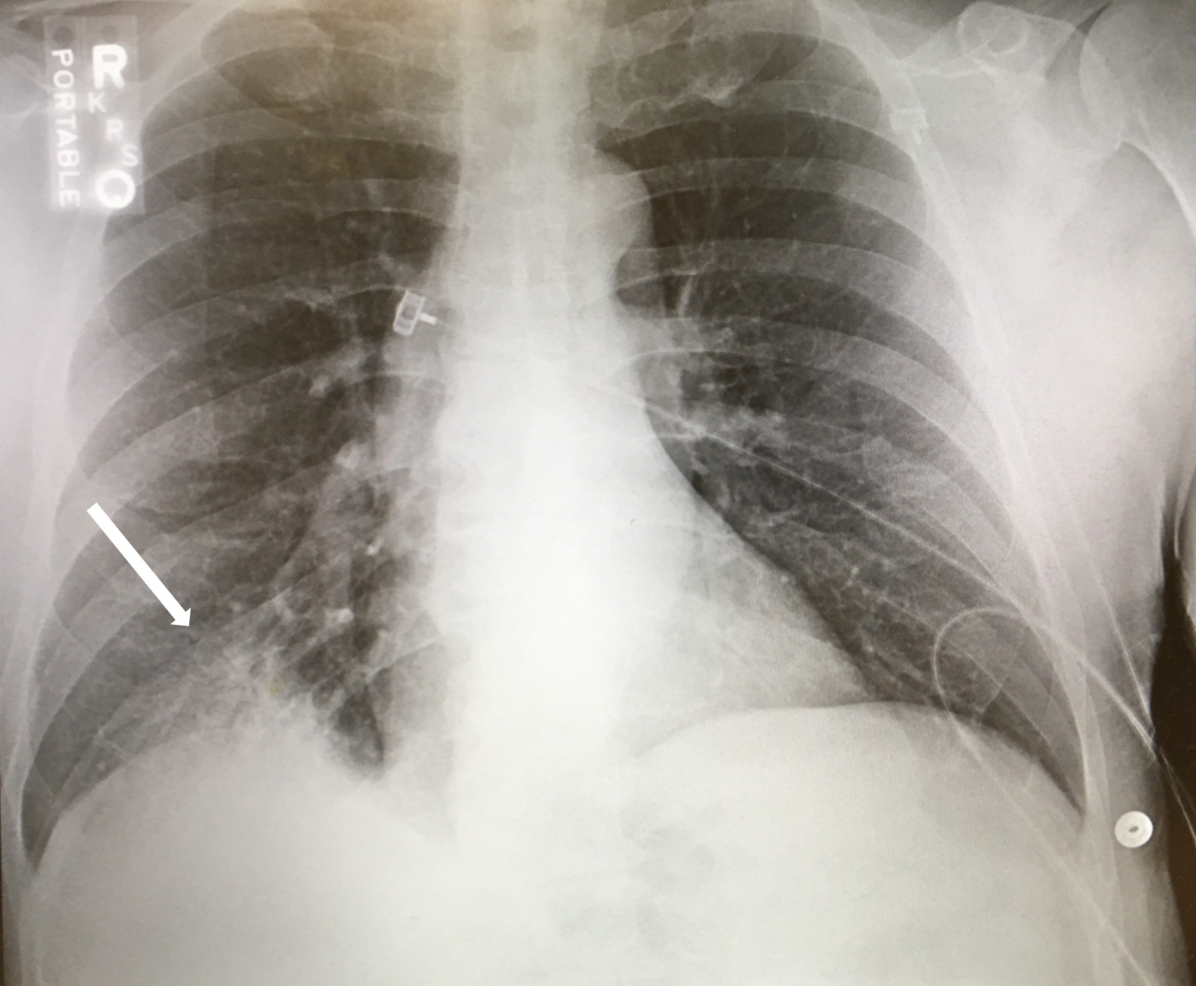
Chest X-Ray Chest X-Ray demonstrating patchy right lower lobe infiltrates.
